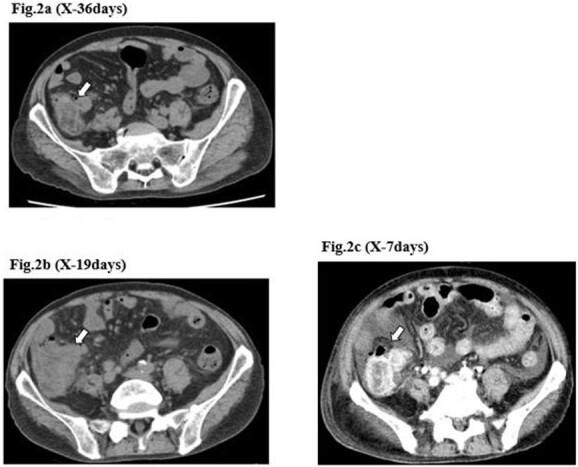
CT image of the ileocecal diverticulitis. The image shows that ileocecal diverticulitis (arrows) has occurred three times (a–c) until the diverticular perforation.
